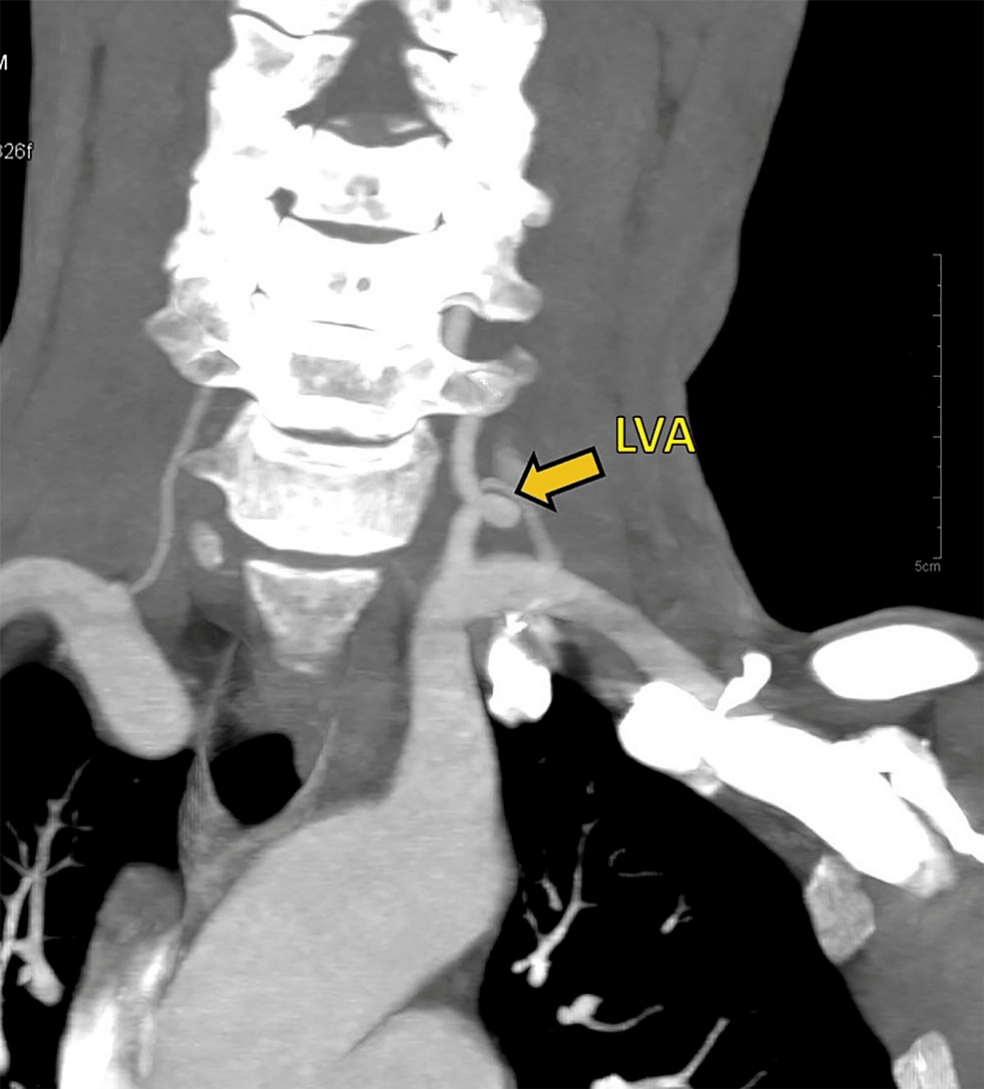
CT angiographic image showing grade III tortuosity of left vertebral artery (yellow arrow, LVA) with a hypoplastic right-sided VA.
